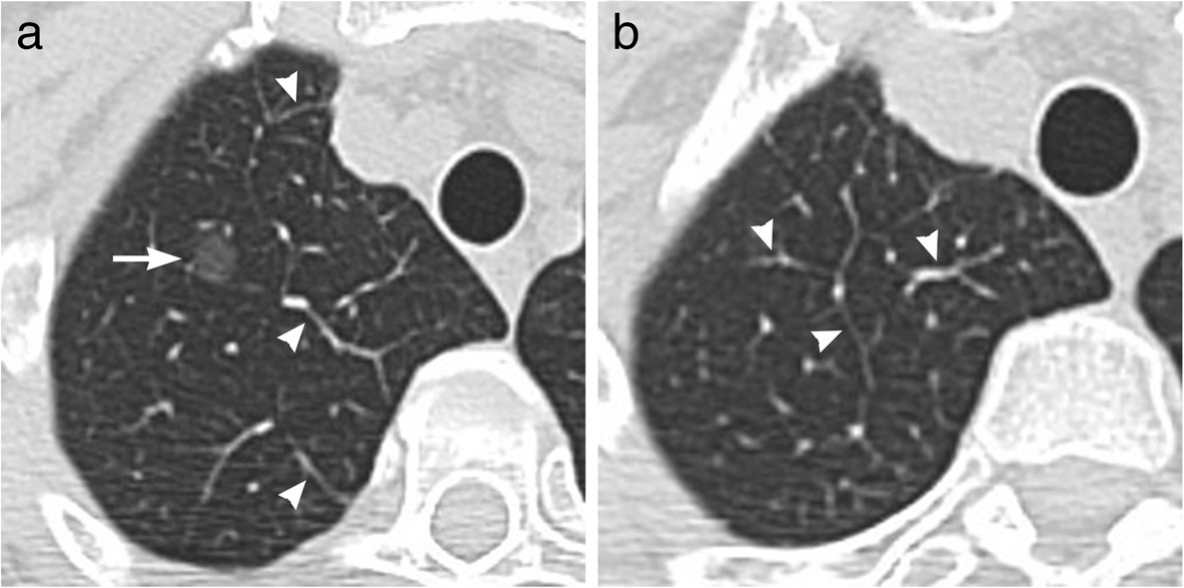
Chest thin-section CT on admission to our hospital. (a) A thin-section CT at the level of right upper lobe shows a 10-mm round, well-defined GGO nodule (*arrow*). There are slightly dilated veins or thickening of the interlobular septa (*arrow heads*). (b) Similar findings are seen also on the slice just above the focal GGO (arrow heads).
